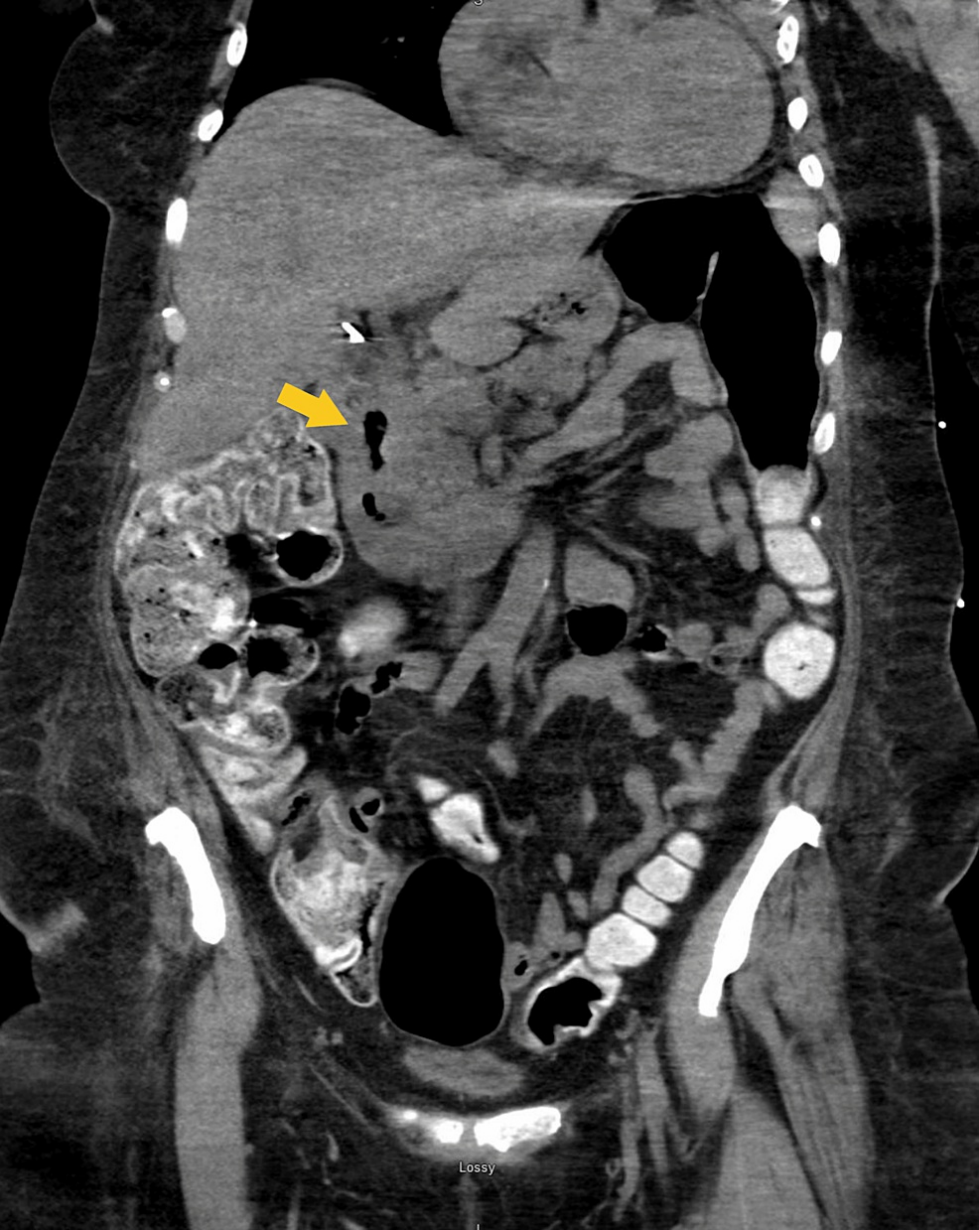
Anteroposterior CT of the abdomen and pelvis after five weeks of chemotherapy sessions
